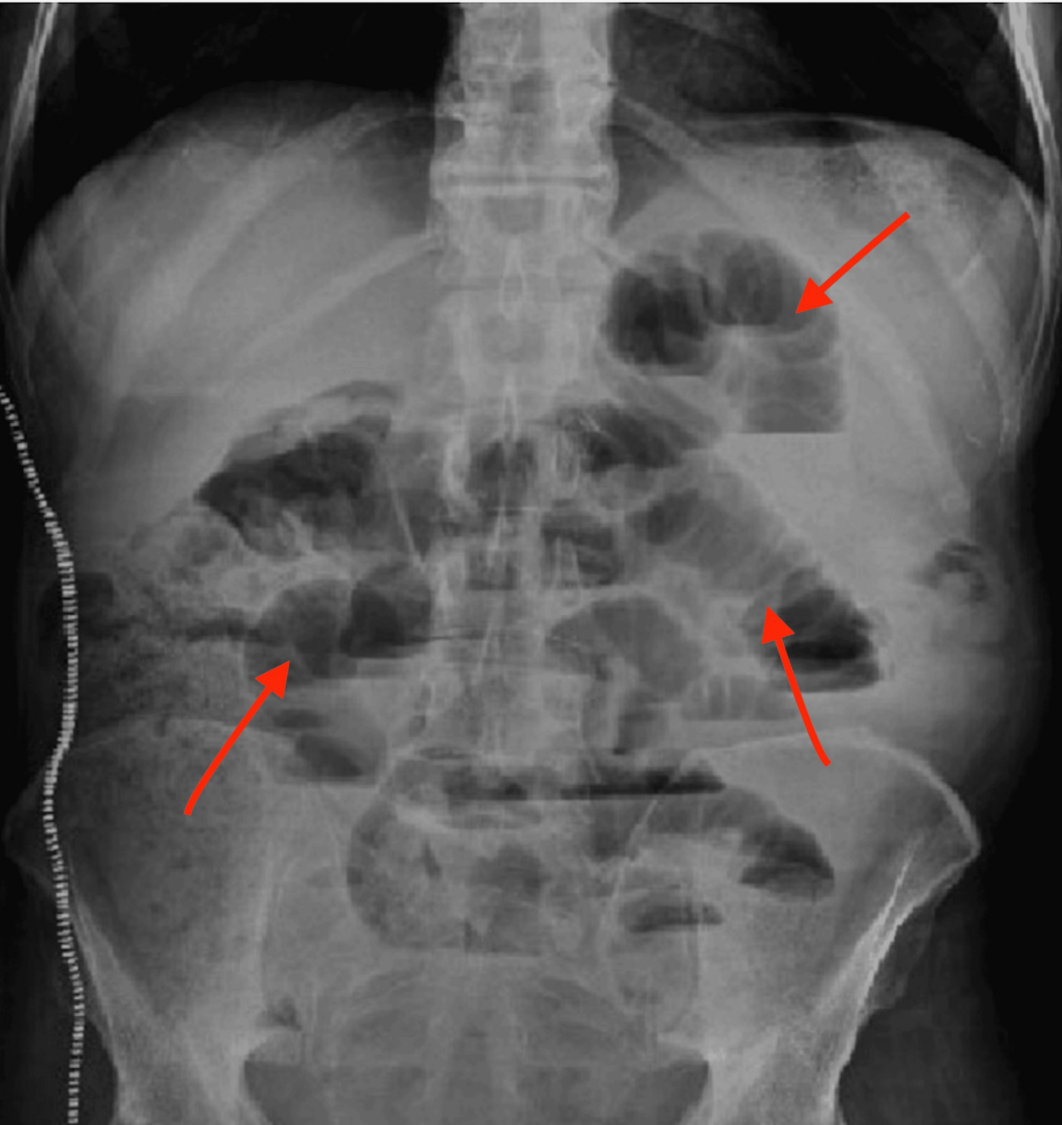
Upright abdominal X-ray showing multiple distended loops of small bowel with air-fluid levels, consistent with partial small bowel obstruction The arrows indicate the areas of bowel dilation.
